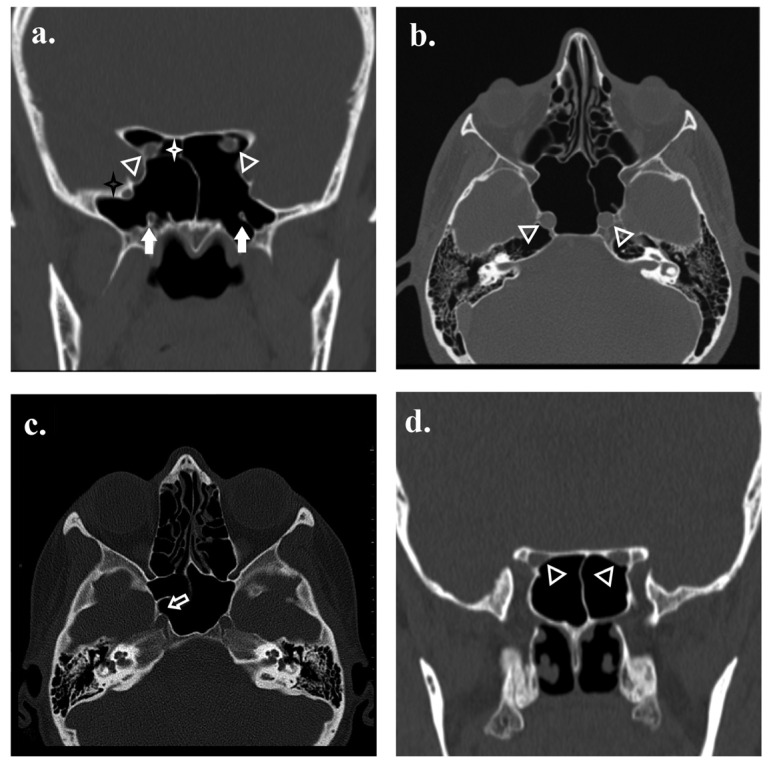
Protrusion and dehiscence of sphenoid sinus–related structures. (a) Coronal CT image demonstrates protrusion of the bilateral optic nerves (arrow heads; anterior angles), right foramen rotundum (black asterisk), and bilateral vidian canals (thick white arrows) into the sphenoid sinus, with the presence of a right spheno-ethmoidal air cell (white asterisk). (b) Axial CT image showing protrusion of the bilateral carotid canals (arrowheads; anterior angles). (c) Axial CT image demonstrating dehiscence of the right carotid canal (arrow). (d) Coronal CT image demonstrating dehiscence of both optic canals (arrowheads; anterior angles).
